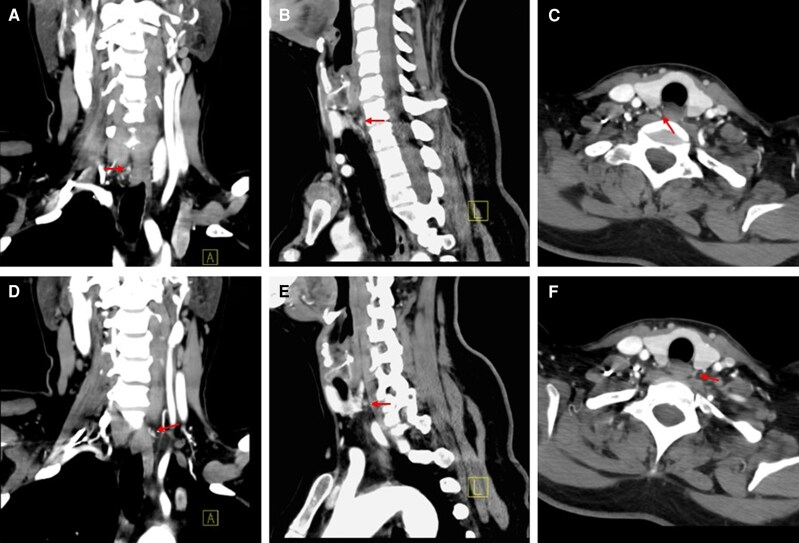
Contrast-enhanced 4-dimensional computed tomography scan showing a 0.5 × 0.2 × 0.3-cm lesion posterior to the inferior pole of the right thyroid lobe in coronal (A), sagittal (B), and transverse (C) views and 1 of similar dimensions posterior to the inferior pole of the left thyroid lobe in coronal (D), sagittal (E), and transverse (F) views.
